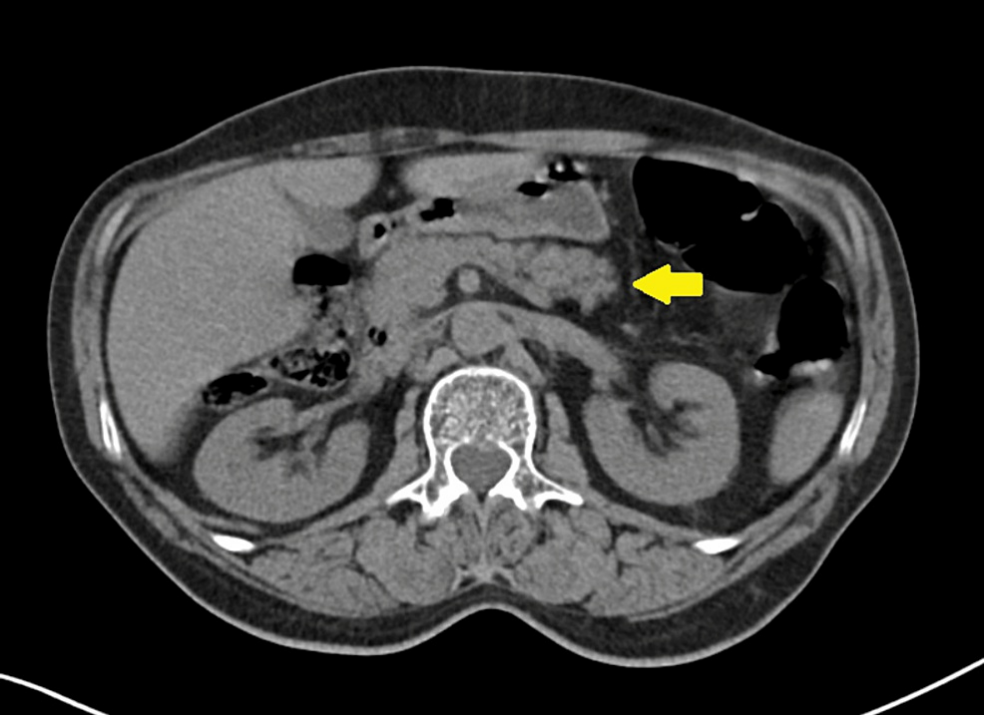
Abdominal computed tomography without contrast demonstrating pancreas without abnormalities (yellow arrow).
